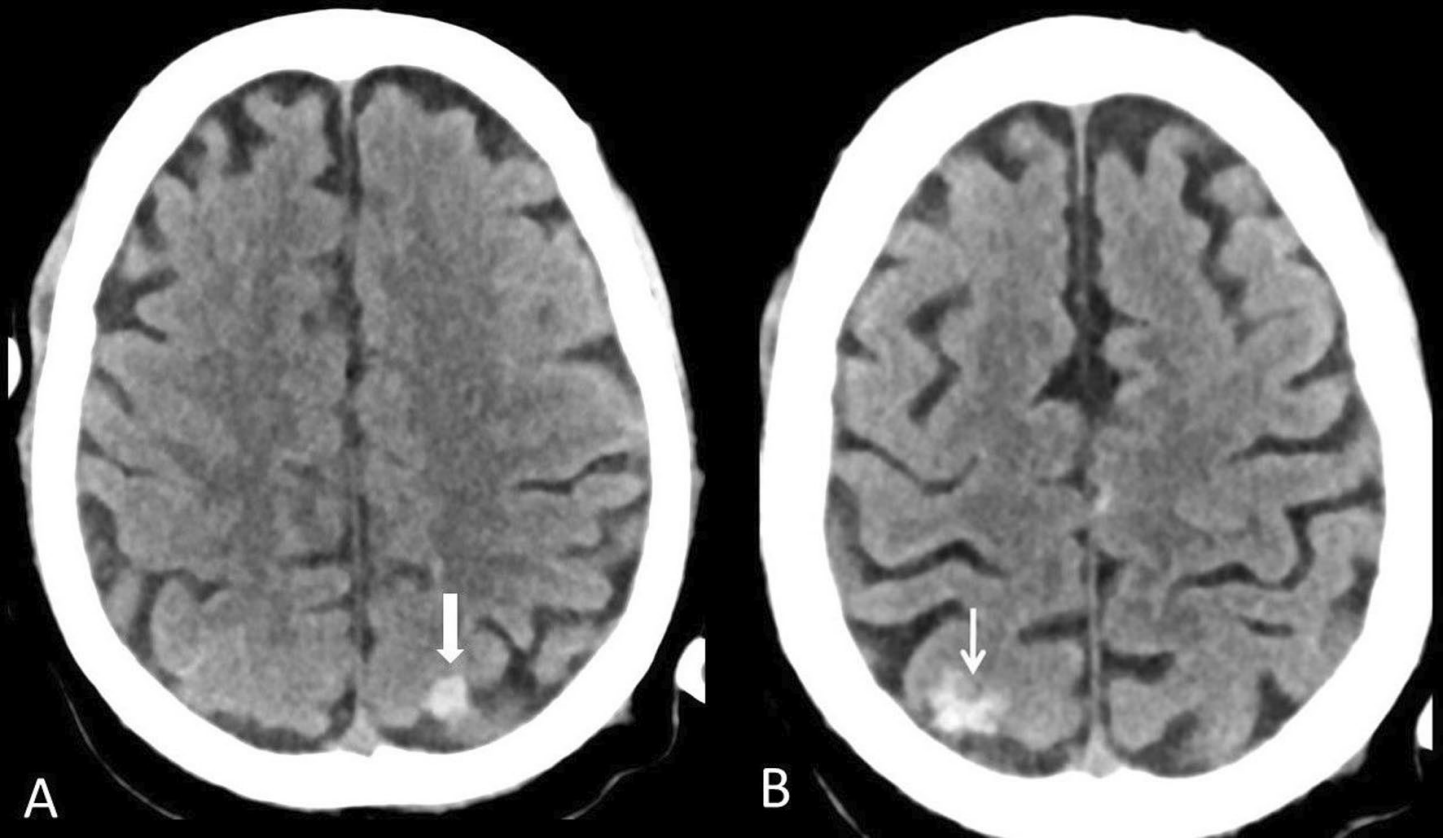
Subarachnoid hemorrhage. 64-year-old man with COVID-19 transferred to ICU and developed acute deterioration of the level of consciousness. a, b Axial CT brain revealed bilateral parietal isolated convexal subarachnoid hemorrhage (arrows)
